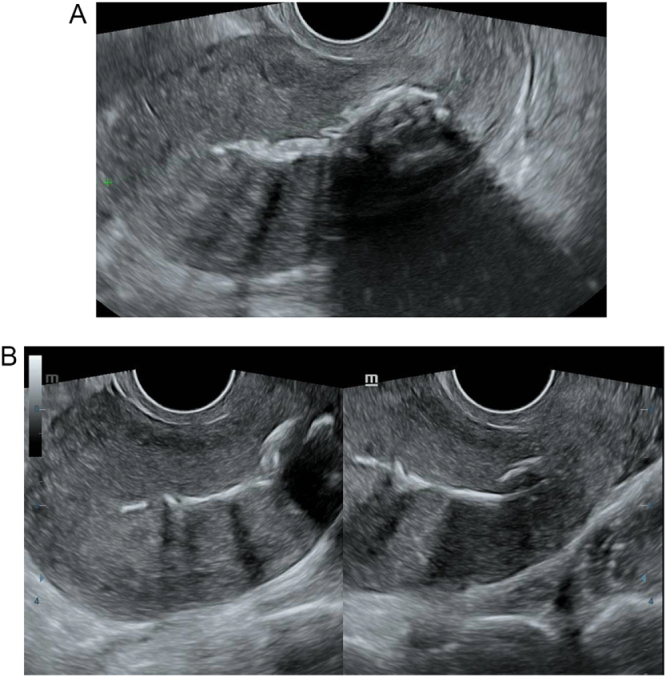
(A) Sagittal transvaginal ultrasound showing hyperechogenic structures within the endometrial cavity with posterior acoustic shadowing, consistent with retained fetal bones. (B) Transvaginal ultrasound transverse views showing hyperechogenic structures within the endometrial cavity with posterior acoustic shadowing, consistent with retained fetal bones.
